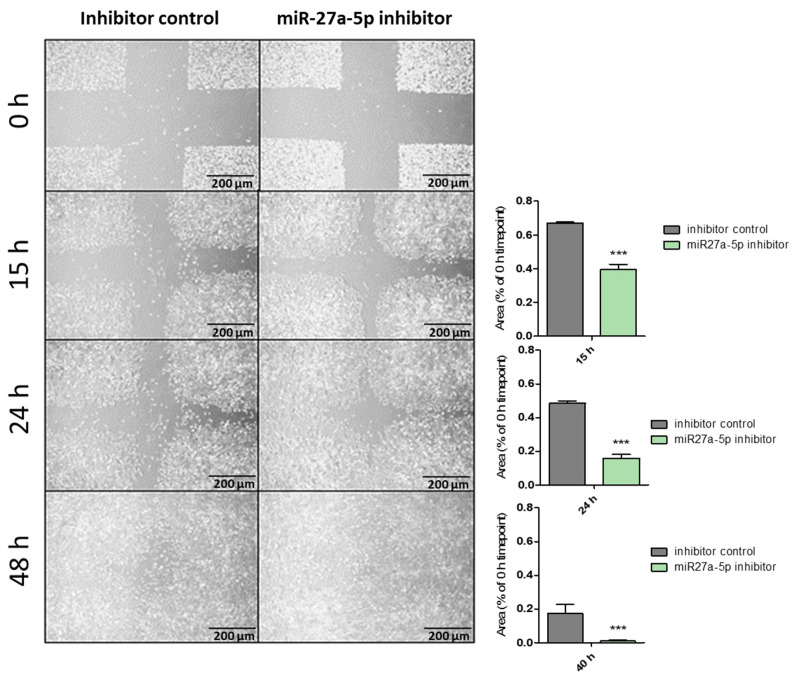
Effect of miR-27a-5p knockdown on cell migration of the triple negative breast cancer cell line SUM159. The area of the scratches was determined using the Image J plugin “MRI Wound Healing Tool” and, for each time point, the remaining area relative to the 0 h (0 h) time point was calculated. Differences between knockdown and control cells were compared by means of one-way ANOVA (* *p* < 0.0001; n = 4).
